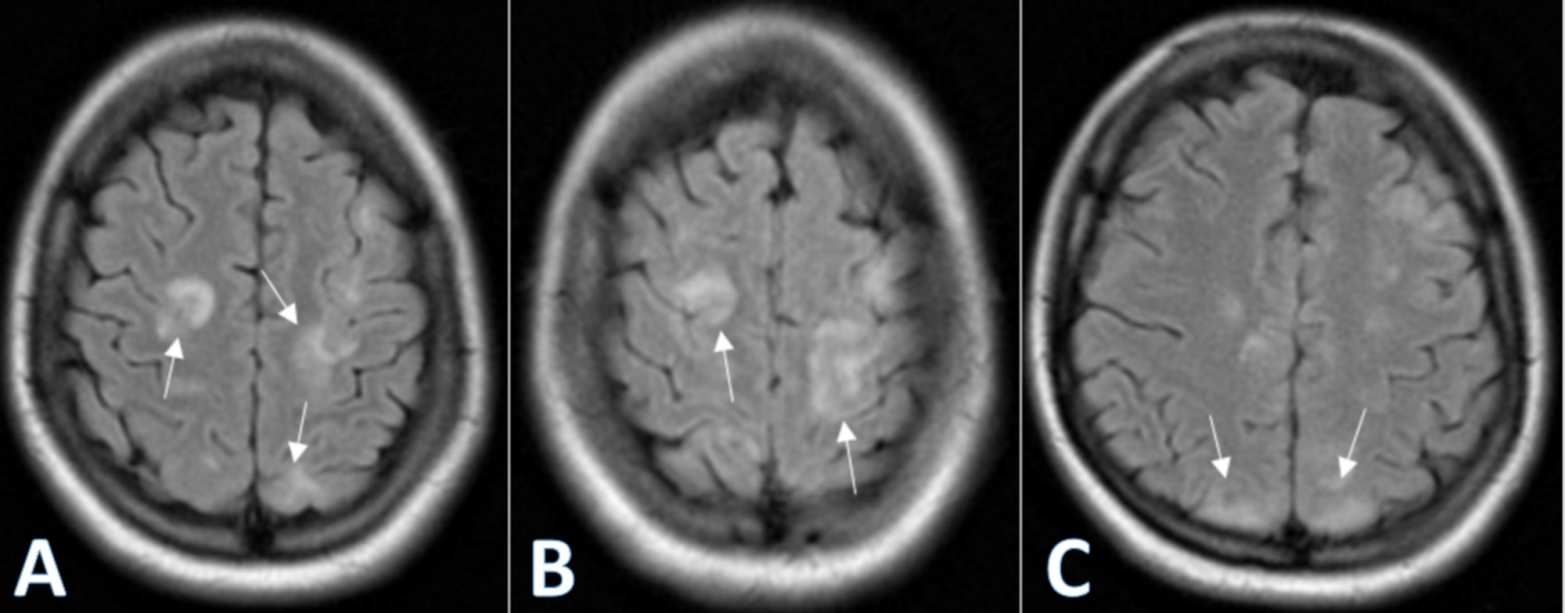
FLAIR imaging of brain MRI demonstrating cortical and subcortical patchy edema with increased signal intensity: (A,B) demonstrate patchy involvement of the parietal, temporal, and occipital lobes (arrows); (C) demonstrates bilateral occipital lobe involvement (arrows) FLAIR: fluid-attenuated inversion recovery
